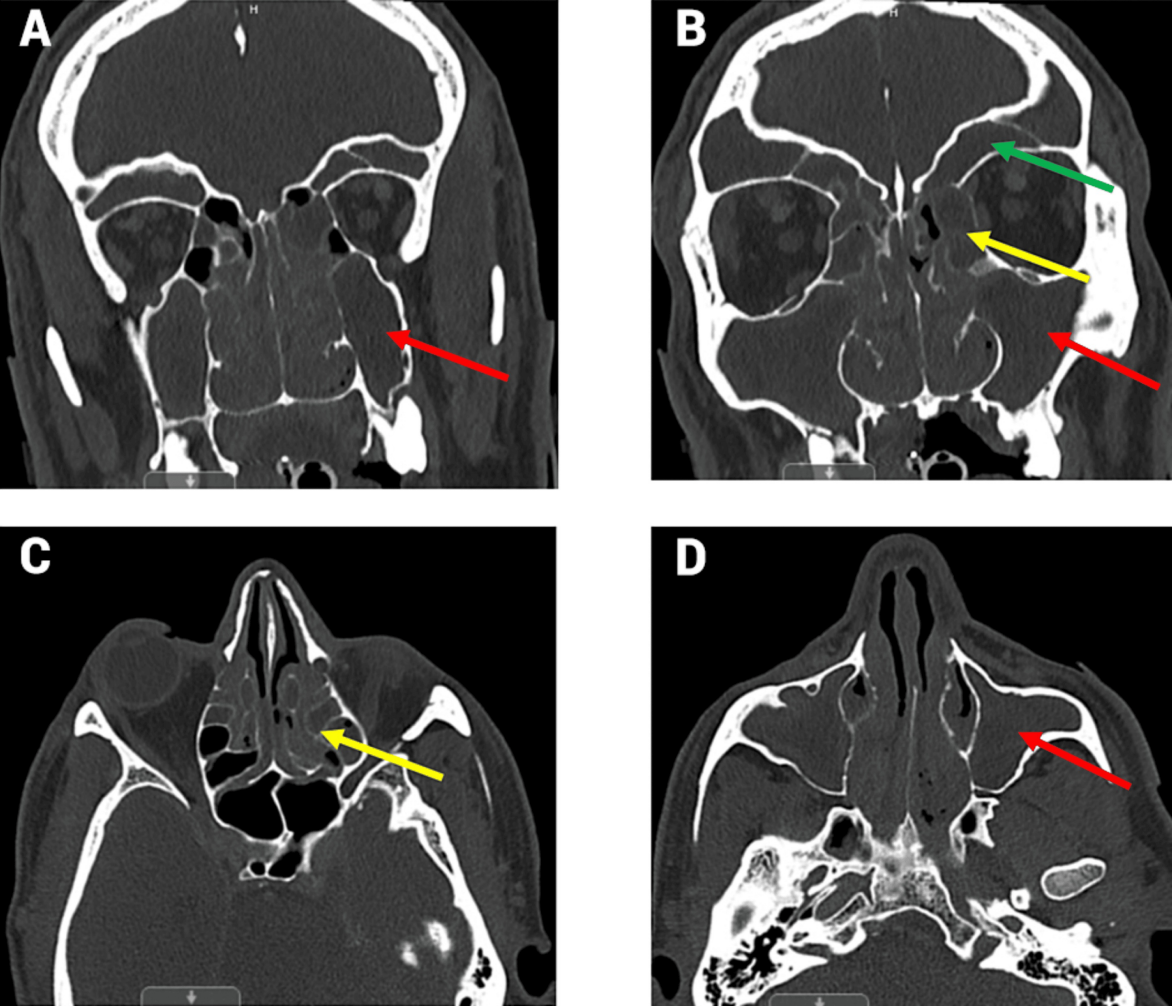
(A, B) Coronal CT images and (C, D) transverse CT images showing extensive sinusitis in the frontal sinuses (green arrow), maxillary sinuses (red arrow), and ethmoid air cells (yellow arrow)
